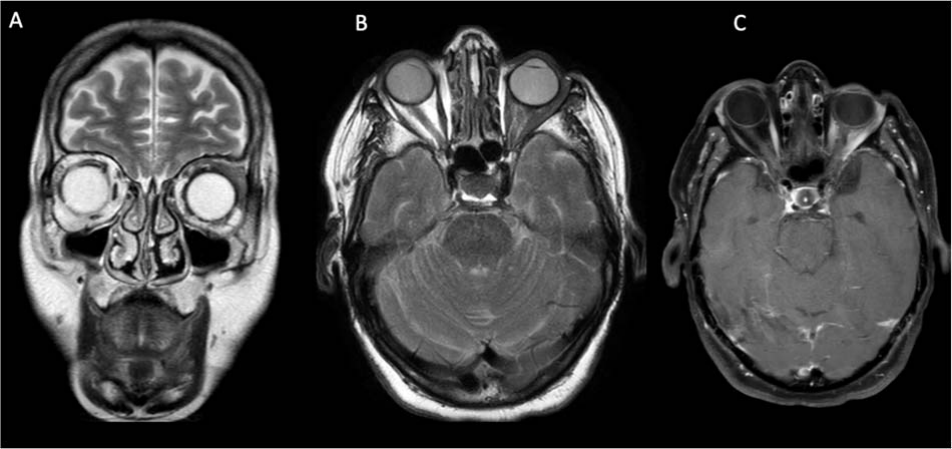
Cerebral and orbital MRI. (A–B) Coronal and axial T2-weighted MRI show iso-intense thickening of the left lateral and superior rectal muscles with fat atrophy. (C) Axial T1-weighted MRI with gadolinium enhancement and fat suppression reveals slight enhancement of the left lateral and superior rectal muscles with infiltration of the surrounding tissues.
